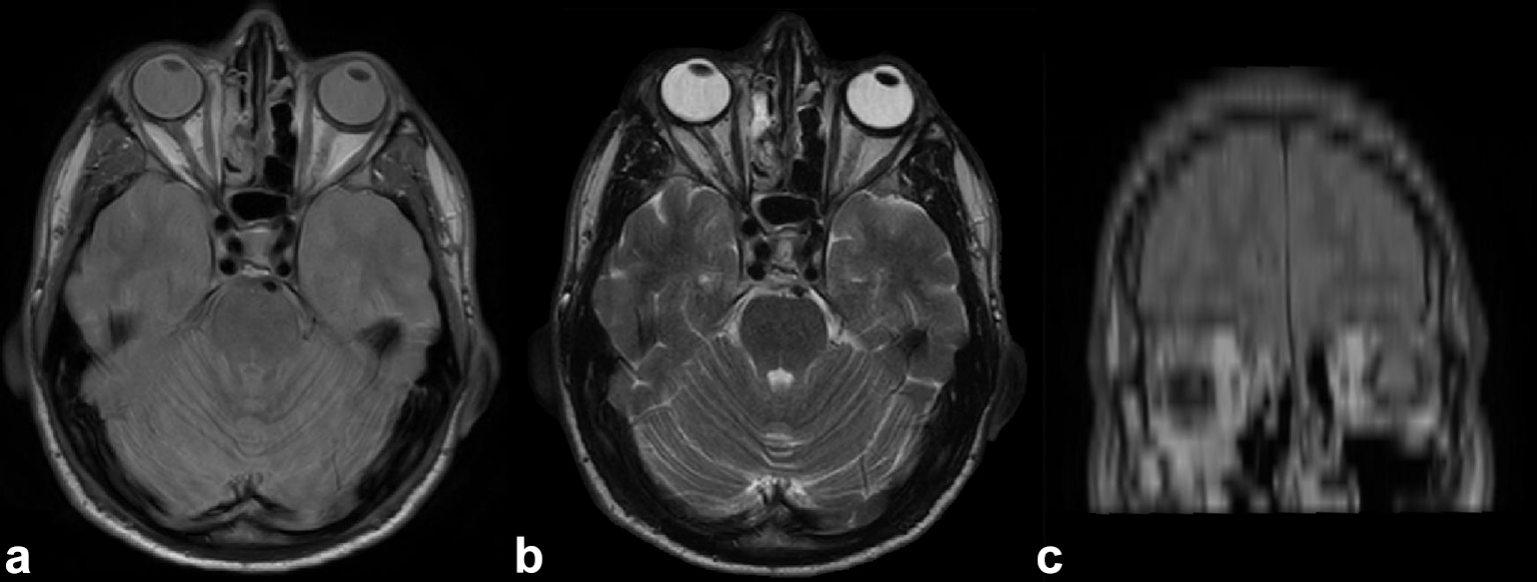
Hypointense *T*_1_ weighted (a), hyperintense *T*_2_ weighted (b) and coronal (c) preoperative MRI images
